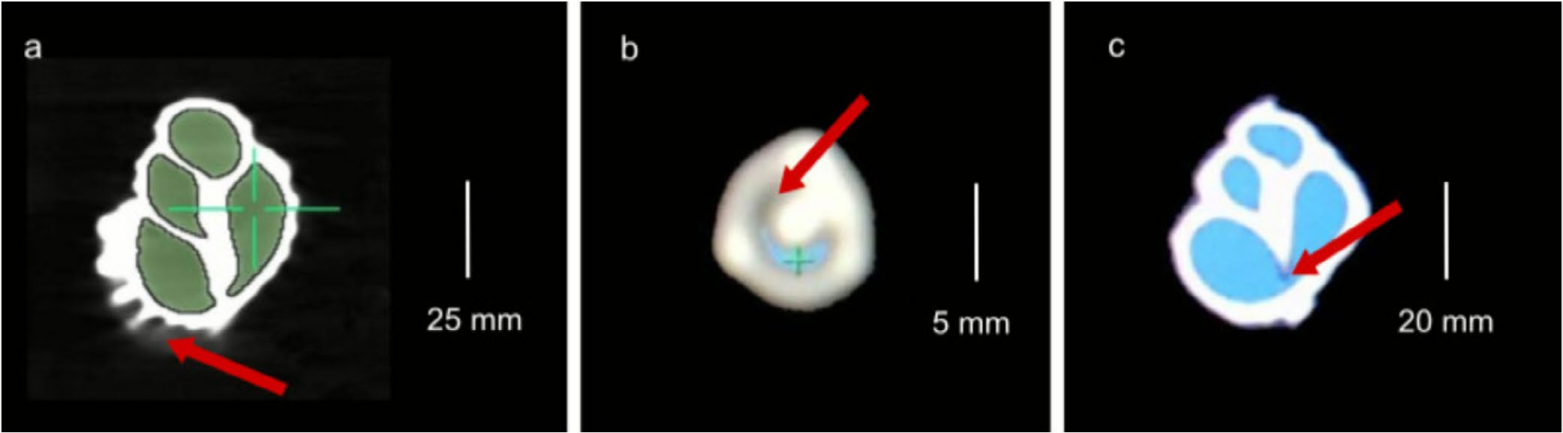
Artifacts in CT images detected in the analyzed images (red arrows). (a) Beam hardening artifact with a false bright in the periphery of sagittal slice from *Monoplex parthenopeus* shell (image from OsiriX MD); (b) Partial volume artifact with a blurring between the shell wall (the “Edge Effect”) in axial slice from
